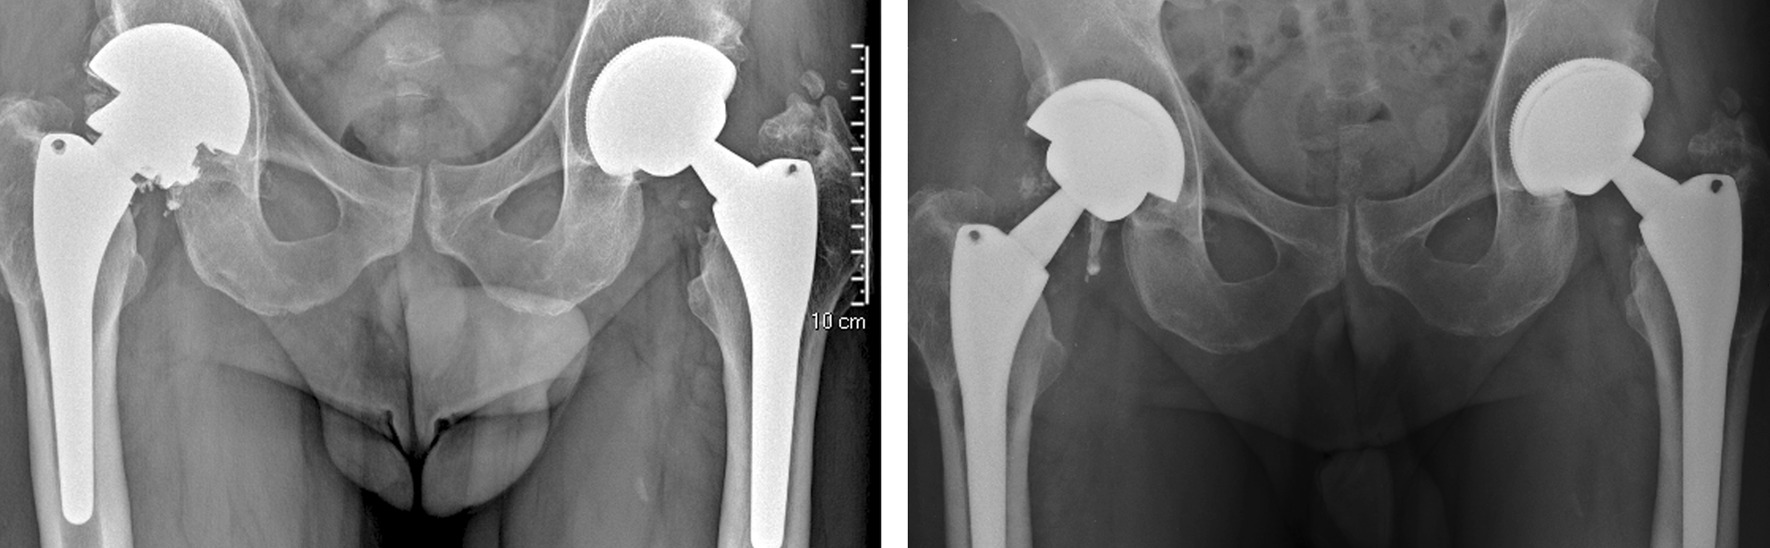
Left: preoperative X-ray showing mechanical failure of ceramic femoral head. Right: postoperative X-ray after components replacement
